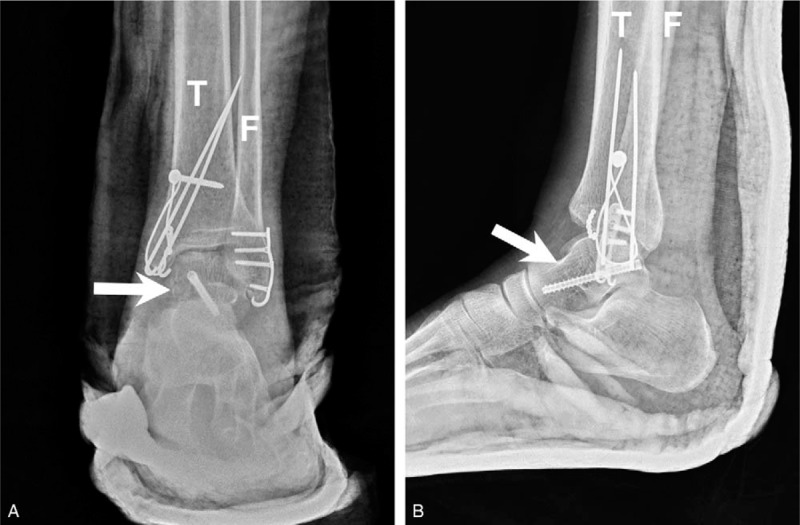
Plain films of the ankle after surgery: anteroposterior and lateral views. Note single screw fixation of the talar fracture. Arrow = talus, T = tibia.
